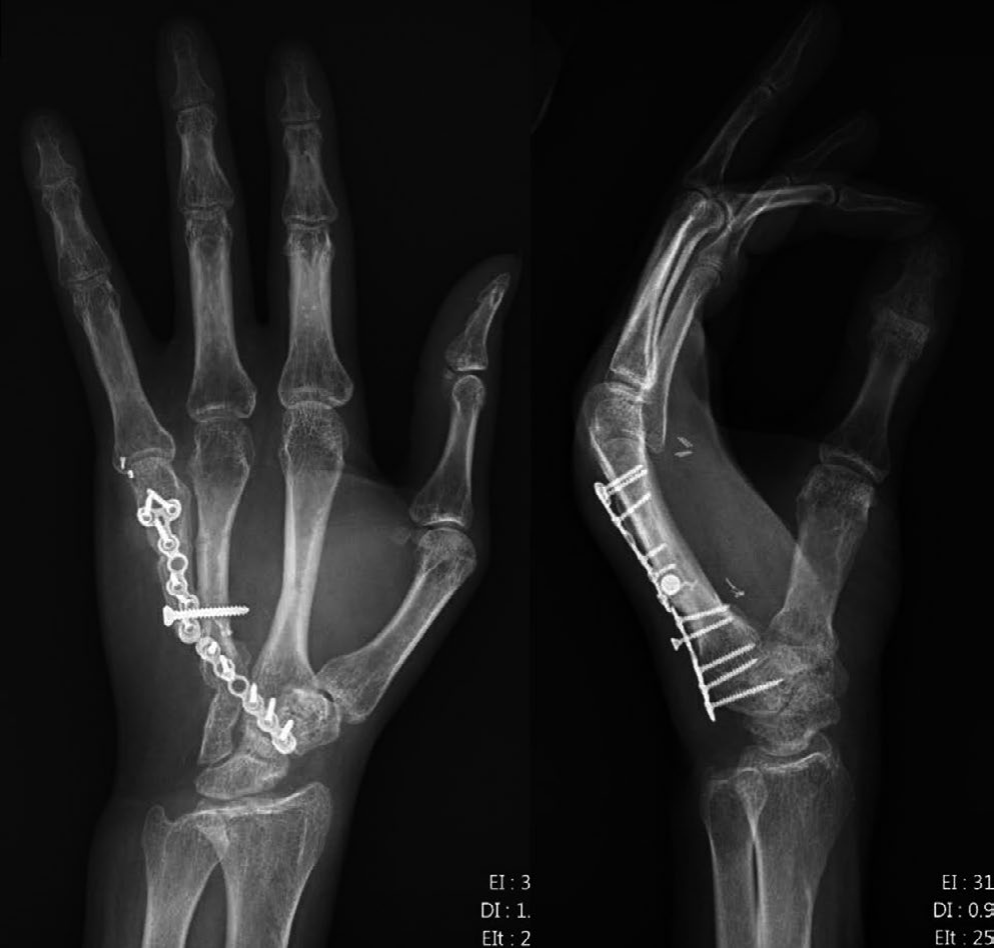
Radiographs taken 28 months after initial resection procedure
